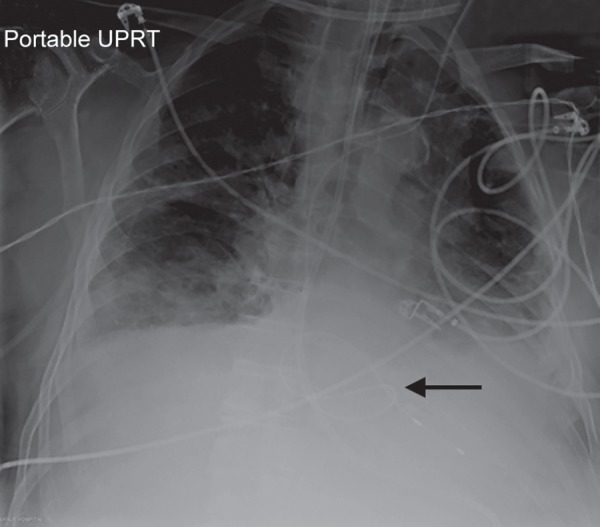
Following TVP insertion, the looped lead is visible in the RV on chest X-ray (arrow)
